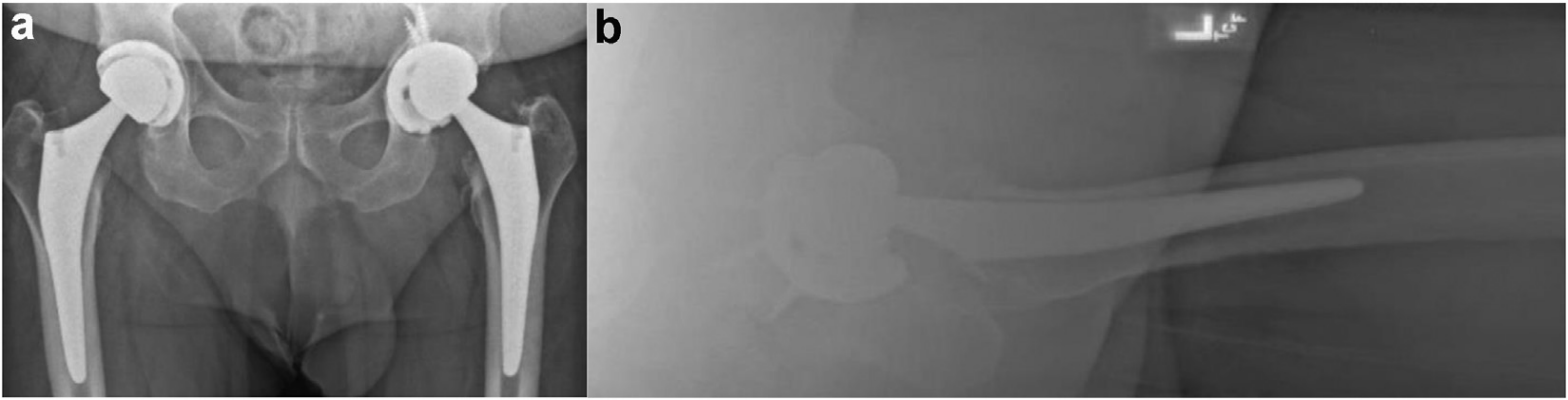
Preoperative left revision THA radiographs. (a) Anteroposterior (AP) pelvis and (b) left lateral radiographs of bilateral THA show the left femoral ball sitting asymmetrically within acetabulum, consistent with dislodgement of the polyethylene liner, 3 years postoperatively. (a) also shows well-fixed right THA without complication, 18 months postoperatively.
